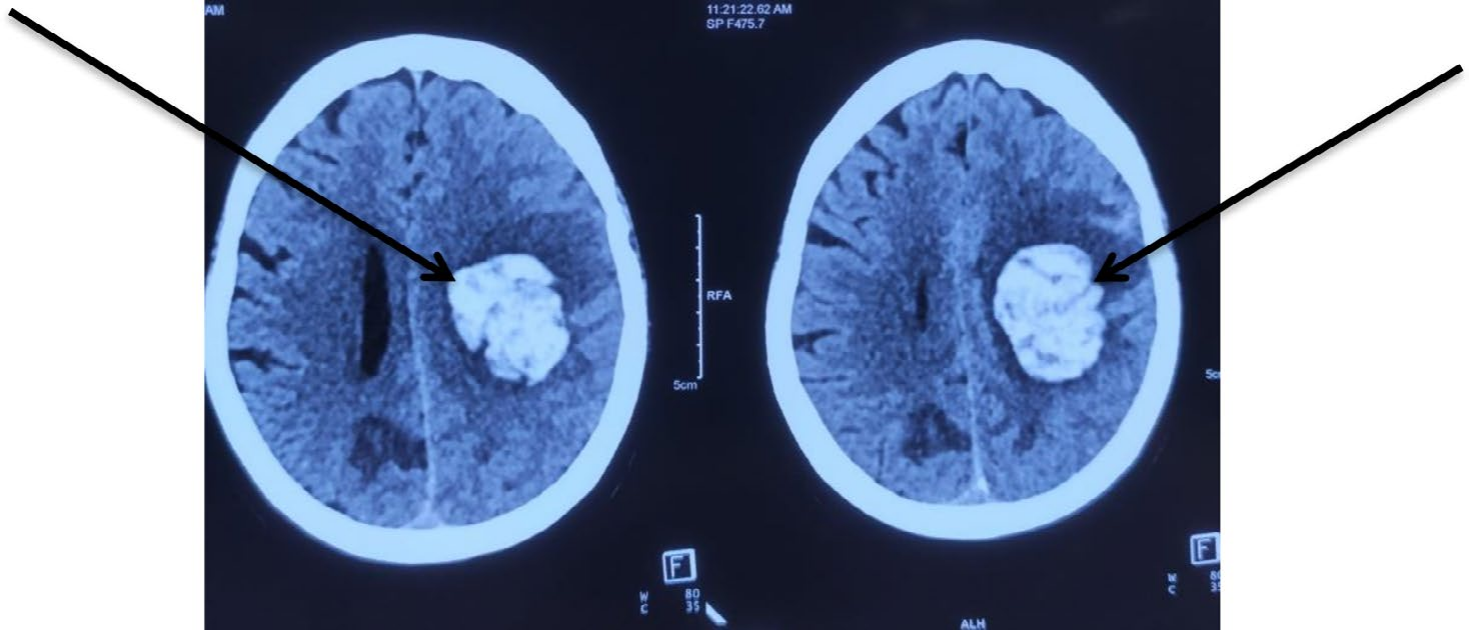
Plain CT head showing hyperdense lesion over left fronto‐parietal region suggestive of ICH (black arrows) measuring around 30 mL.
